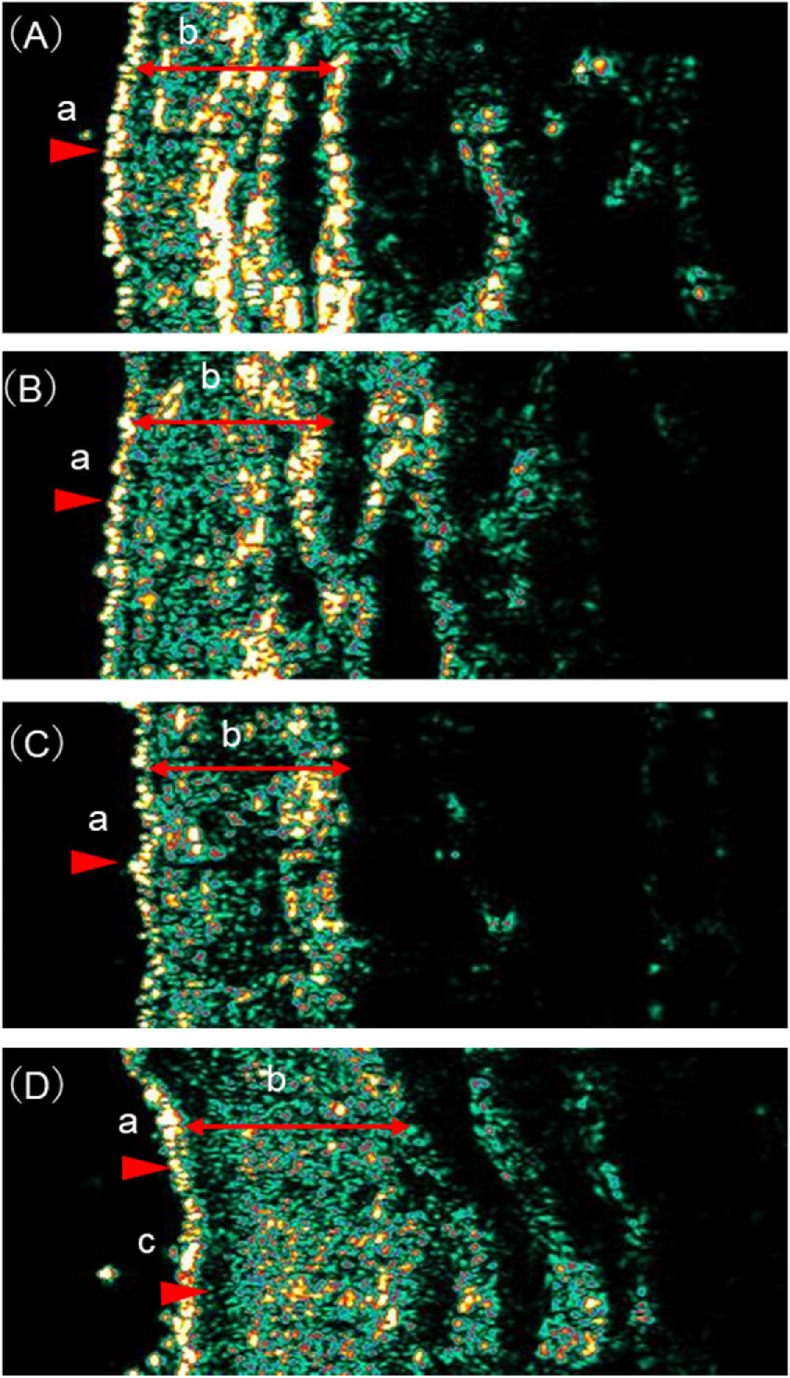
Representative ultrasonic images. One representative case from each group is provided: “erythema – pigmentation” in the non-severe group (A), “erythema–keratin peeling” in the non-severe group (B), “localized erosion–epithelialization” in the severe group (C), and “widespread erosion–crusting” in the severe group (D). The color scale of echogenicity is white-yellow-red-green-blue-black. a, epidermis, b, dermis, c, zonal hypoechoic areas in papillary dermis (SLEB grade 3). (C) and (D) Lower dermis echogenicity than (A) and (B) (*P* ​= ​0.036). Only (D) has a SLEB grade of 3. SLEB, subepidermal low echogenic band.
